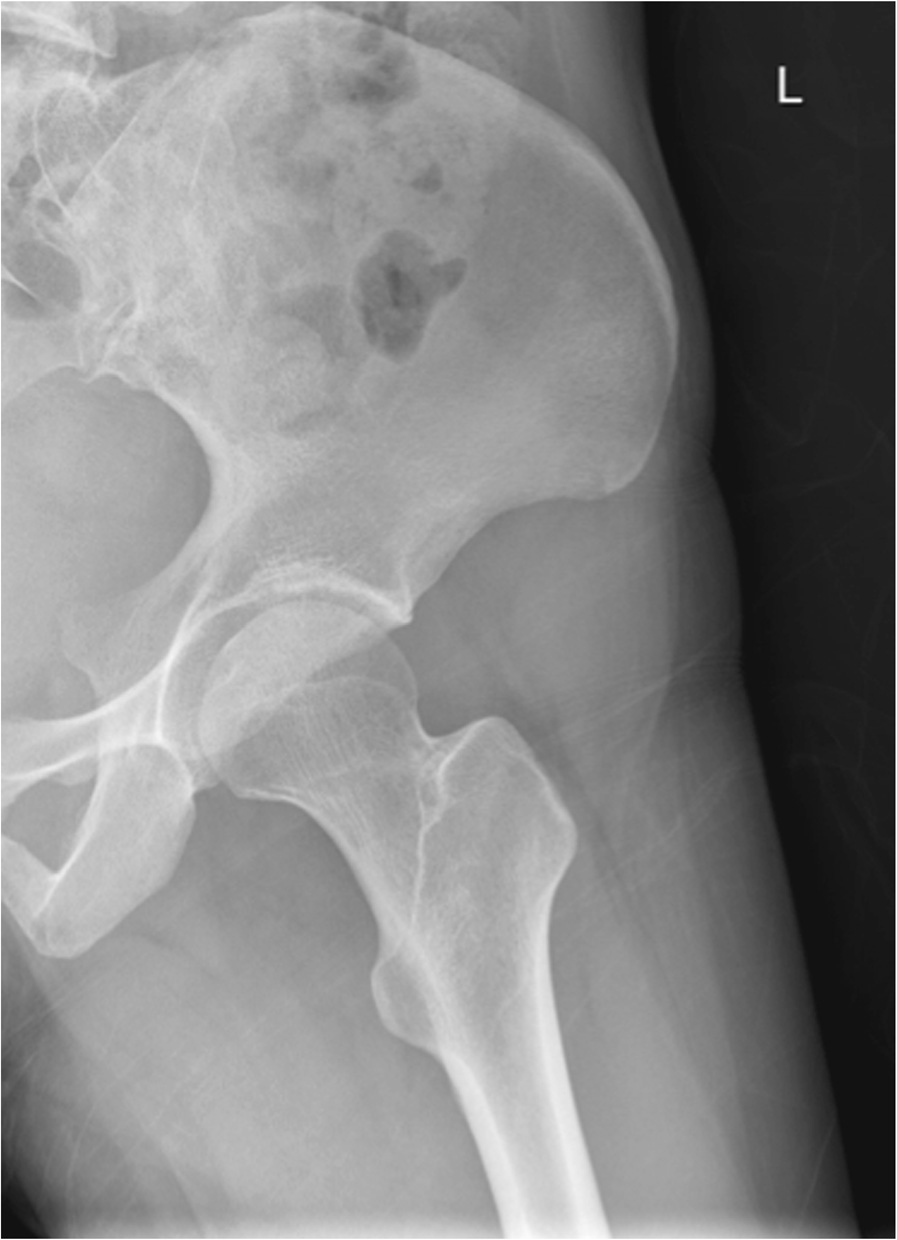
Normal X-ray left hip - Lateral view.
